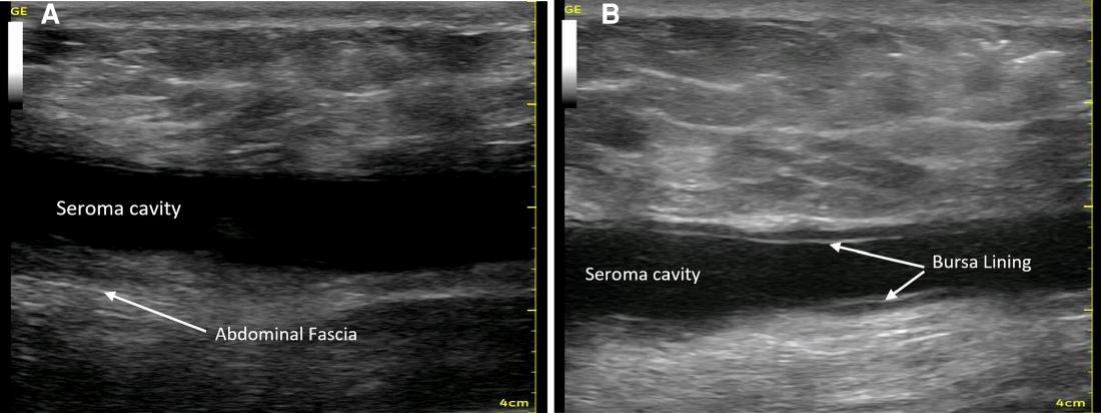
(A) Typical seroma appearance on high-resolution ultrasound (HRUS), prior to the use of Interi (Internal Closure System, IC Surgical, Grand Rapids, MI), showing ∼200 cc of fluid 3 weeks following an abdominoplasty with prior standard of care Blake drain. (B) Seroma cavity after partial drainage with evidence of a pseudocyst lining. Video 2 shows ultrasound-guided drainage.
